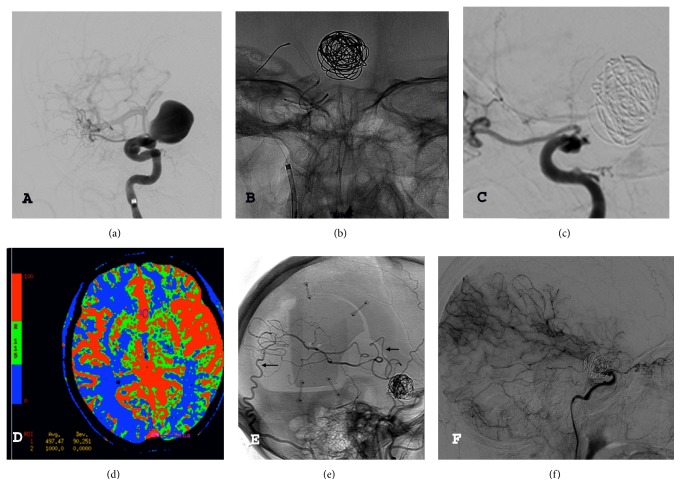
(a) Digital subtraction angiography showing a giant carotid-ophthalmic aneurysm on the right side. (b) Coiling and flow diverter deployment. (c) Digital subtraction angiography showing the occlusion of the internal carotid artery on the right side. (d) Perfusion-weighted CT scan: reduced cerebral blood flow on the right hemisphere. (e-f) Postoperative digital subtraction angiography (early and late phases) showing the patency of the double-barrel bypass (arrows) and the territory perfused by the bypass.
